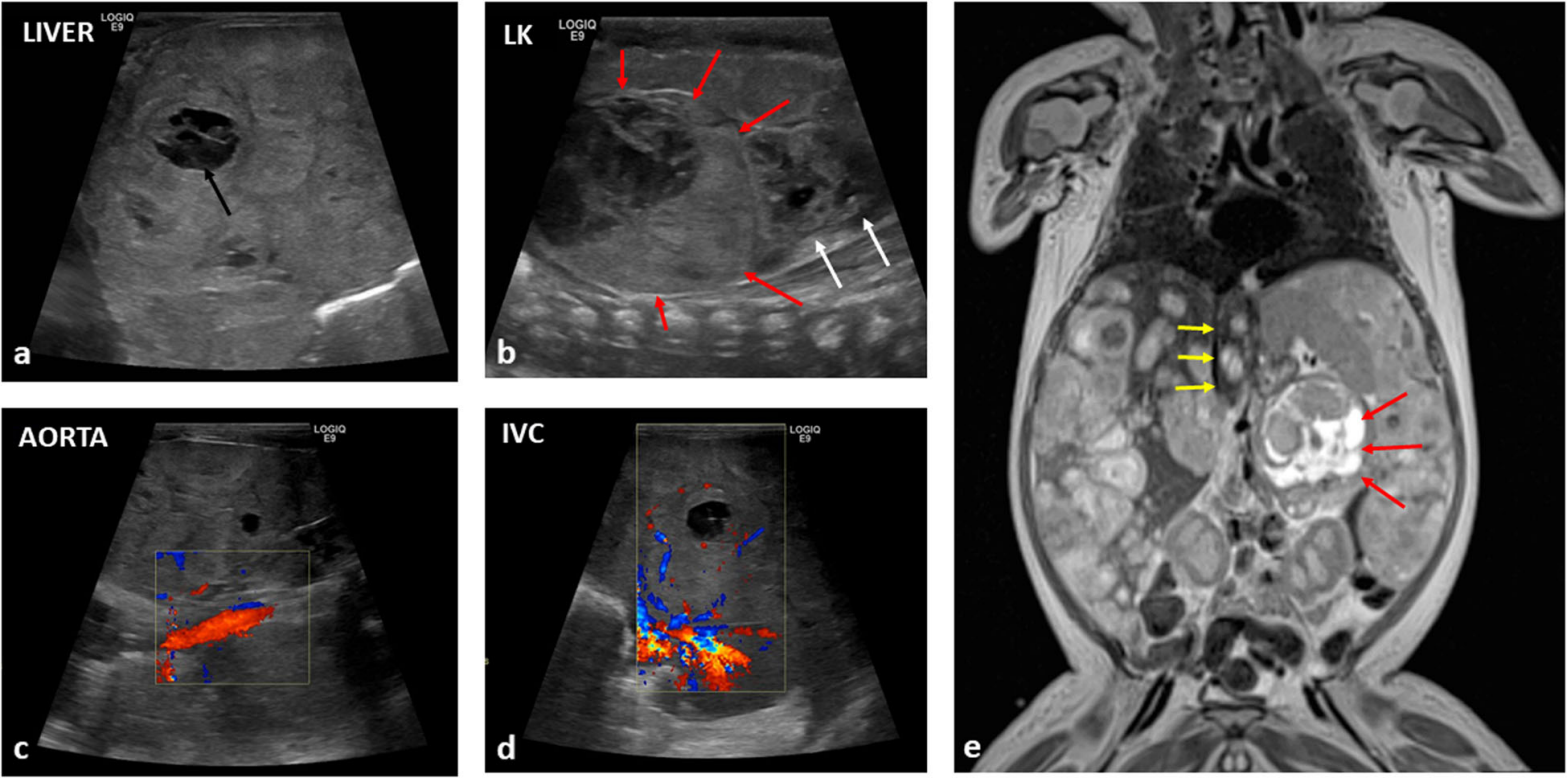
A newborn boy diagnosed with high risk, neuroblastoma at birth, presenting with abdominal distension, vomiting and discomfort. a Longitudinal ultrasound image of the right lobe of the liver demonstrates heterogenous internal echotexture with the impression of numerous mass-like lesions, some appearing necrotic (black arrow). b A longitudinal view of the left flank reveals a large suprarenal mass (red arrows), causing inferior displacement of the left kidney (white arrows). c Longitudinal views of the aorta demonstrates patency, although those of the (d) inferior vena cava only reveal scant flow within the liver. e Coronal T2-weighted MR imaging subsequently confirms the findings, demonstrating the left suprarenal mass (red arrows) with multiple diffuse metastases within the liver. The upper abdominal inferior vena cava is compressed (yellow arrows) putting the patient at risk of abdominal compartment syndrome. The patient also suffered from respiratory compromise from the enlarged liver hindering diaphragmatic movement, hepatic failure, obstructive jaundice and coagulopathy. As a secondary consequence of the large tumour bulk at diagnosis, the patient was also treated for tumour lysis syndrome after initiation of chemotherapy
